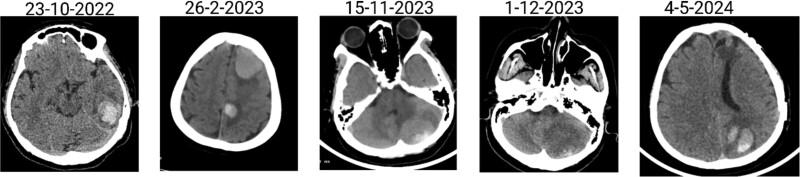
Brain CT scan showing multiple previous haemorrhagic lesions in the brain parenchyma with evidence of chronicity.
